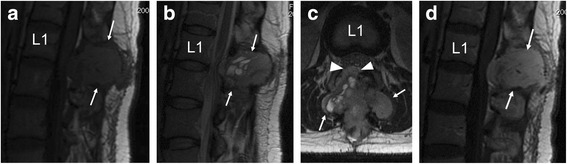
Preoperative magnetic resonance (MR) imaging. a Sagittal T1-weighted MR image shows an extradural tumour exhibiting mild hyperintensity in the L1 vertebral arch (*arrows*). b Sagittal T2-weighted MR image shows a mass with heterogeneous hyperintensity (*arrows*). c Axial T2-weighted image reveals a posterior epidural component of the L1 arch lesion (*arrows*), with compression of the thecal sac (*arrow heads*). d Sagittal gadolinium-enhanced MR image reveals a well-enhanced tumour in the L1 vertebral arch (*arrows*). Abbreviation: *L1* body of the first lumbar vertebra
